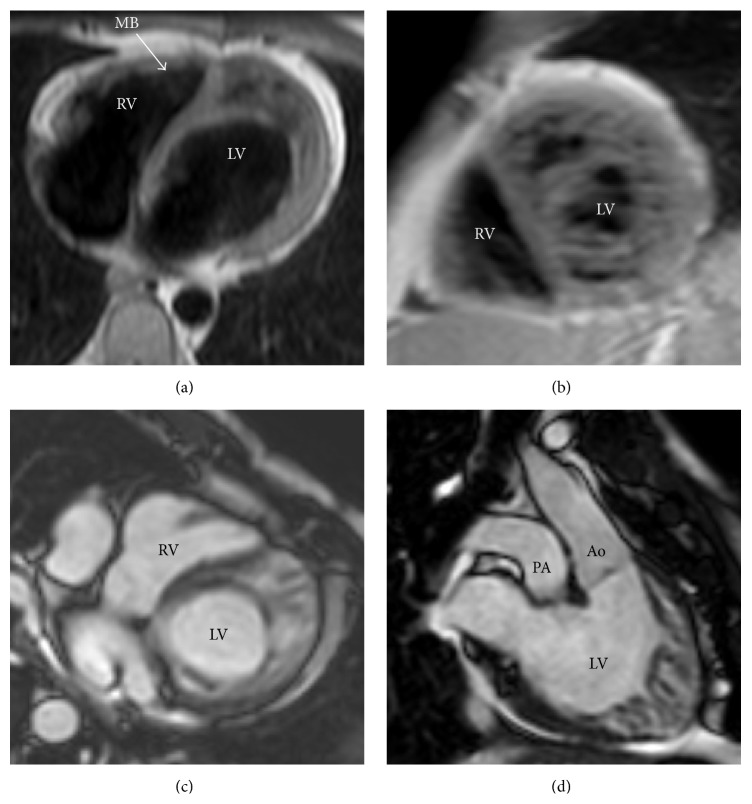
Cardiac MRI long axis (a) and short axis (b) black blood fast spin echo images of LV, with end-diastolic long axis ((c), (d)) cine balanced steady-state free precession acquisition, confirming the presence of the anatomic features of NC in the mid and apical segments. MB moderator band (arrow).
